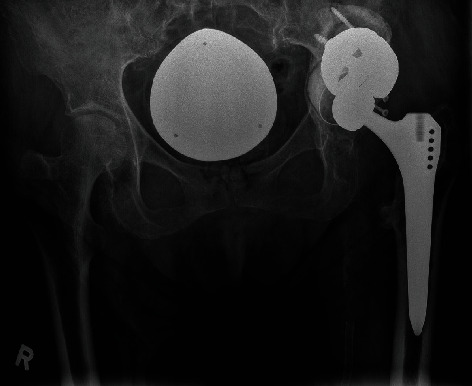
Last X-ray of the patient in clinic after the cemented cup revision. No further episodes of left hip dislocation.
